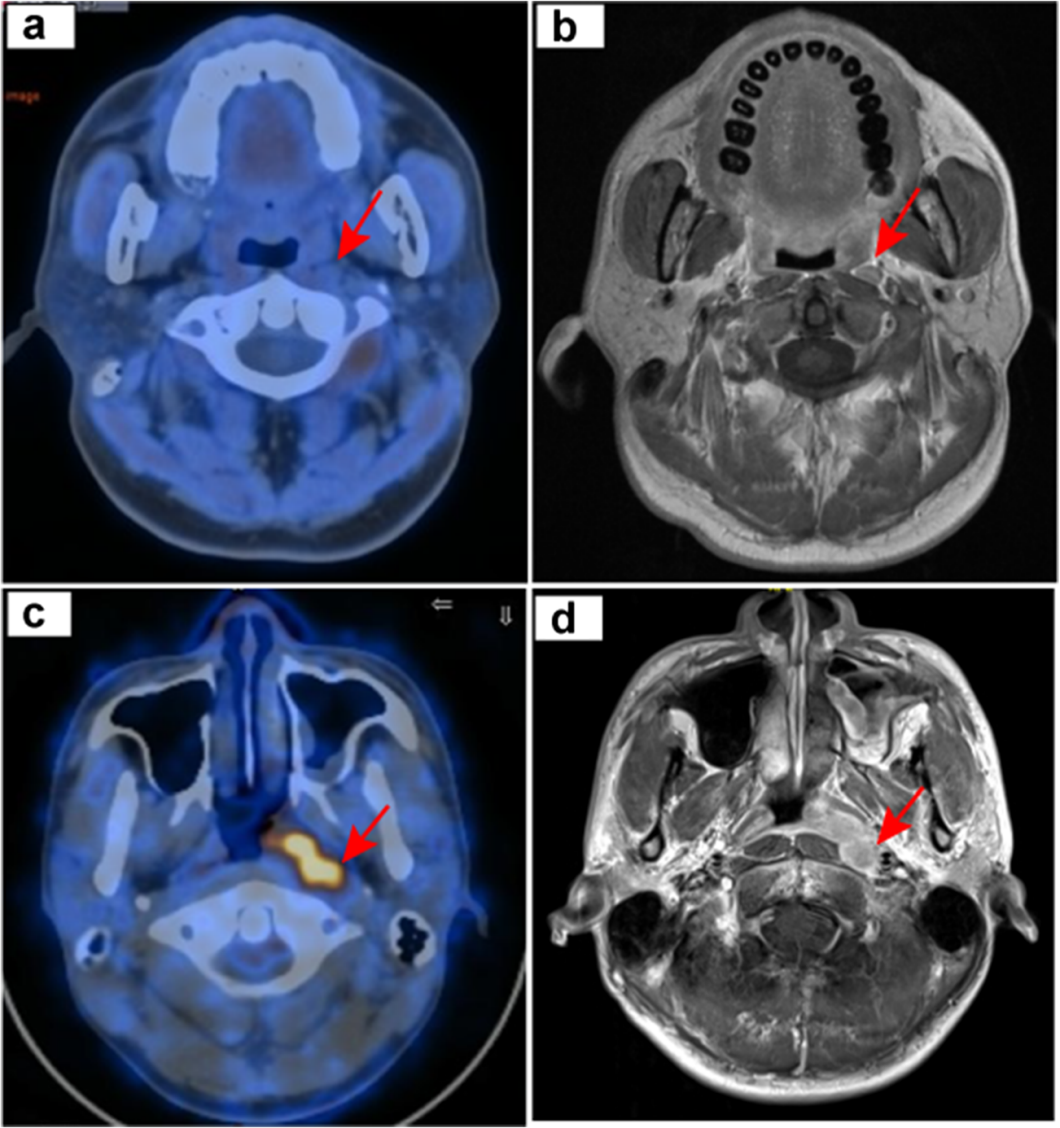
Detecting discrepancies of retropharyngeal and neck lymph nodes between PET/CT and head-and-neck MRI. (a, b) Male, 45 years old, T2N1M0 NPC. PET/CT image (left). Contrast-enhanced T1-weighted MRI image (right). Metastatic retropharyngeal lymph node was detected by MRI (red arrow) but not PET/CT. (c, d) Male, 50 years old, T2N1M0 NPC. PET/CT image (left). Contrast-enhanced T1-weighted MRI image (right). Metastatic retropharyngeal lymph node was obvious on MRI (red arrow), while it disappeared on PET/CT because of the shadow of the primary lesion
